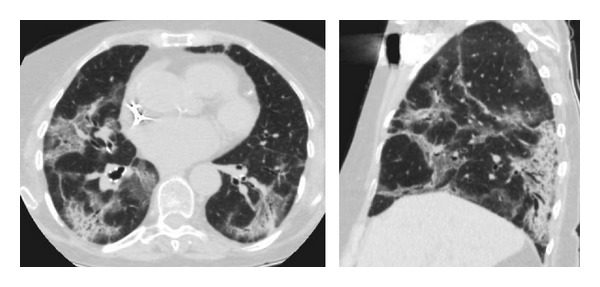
Drug induces lung changes with the use of amiodarone in an 81-year-old patient. Computed tomography shows the pattern of cryptogenic organizing pneumonia.
